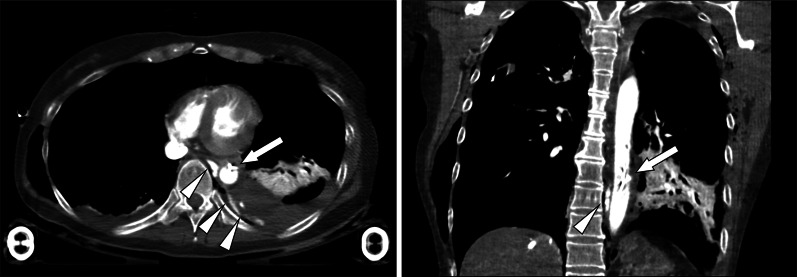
Computed tomography findings after aortic cross-clamping. Contrast-enhanced computed tomography showing an endovascular balloon in the thoracic aorta (arrow) and extravasation of contrast medium adjacent to the descending aorta (arrowhead)
